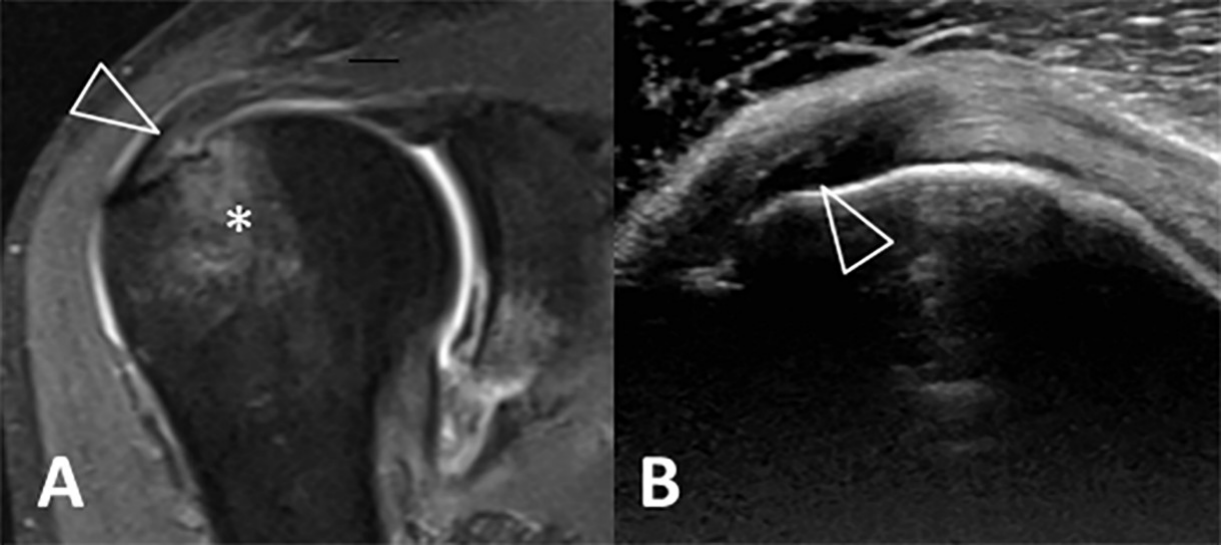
Coronal fat saturated proton density MRA (A) and Ultrasound (B) of the right shoulder of a 53-year-old male patient. There is an articular-sided hyperintense signal change at the supraspinatus tendon insertion (A, open arrow); on the corresponding ultrasound image of the same patient, there is an area of low echogenicity at the same location of the tendon (B, open arrow). In addition, extensive bone marrow edema in the humeral head is seen on MRA (A, asterisk). Additional finding of thickening and narrowing of the axillary recess on the MRA raises the possibility of adhesive capsulitis.
